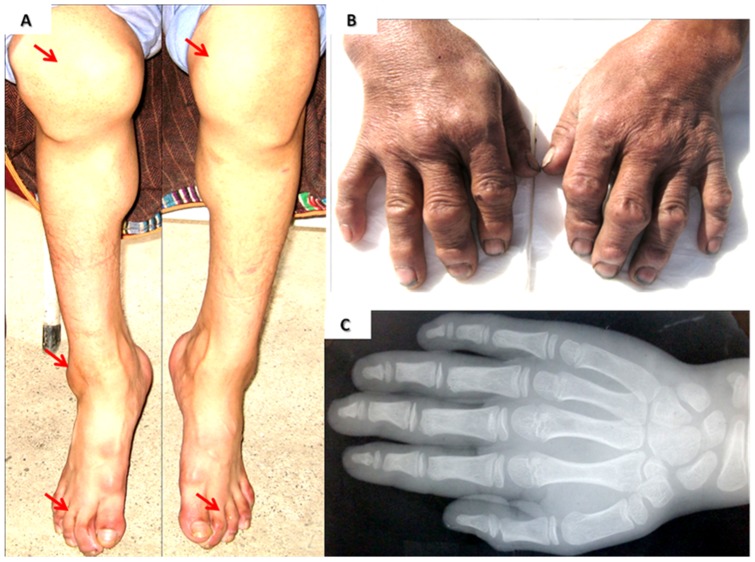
Clinical features of KBD patients. A. A female KBD patient with her knee, ankle and toe joints deformity, the arrows show multiple joints of this patient are affected. B. A male KBD patient with his fingers joints deformity. C. The X-ray picture of a KBD patient's hand.
